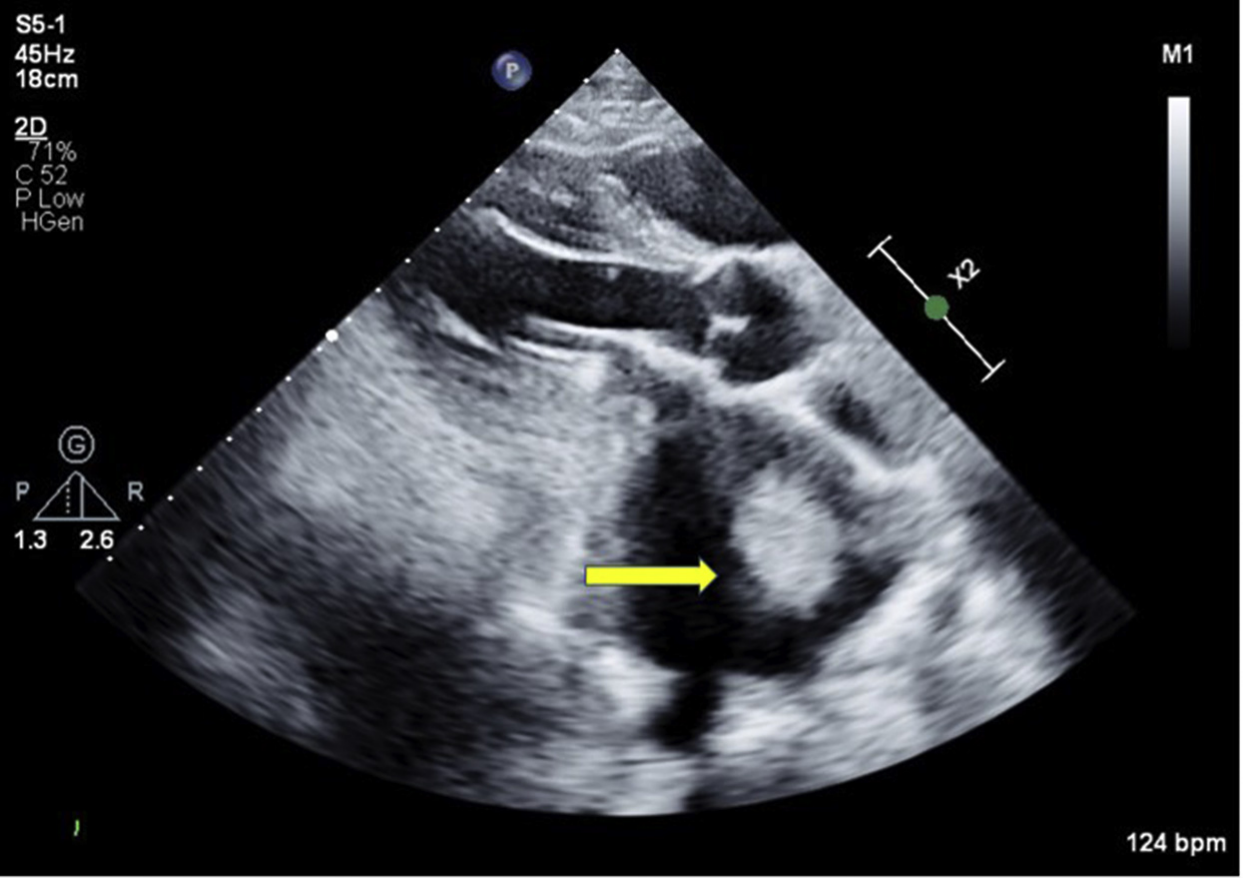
Transthoracic echocardiography: two-dimensional parasternal long-axis view showing LA mass (*yellow arrow*).
